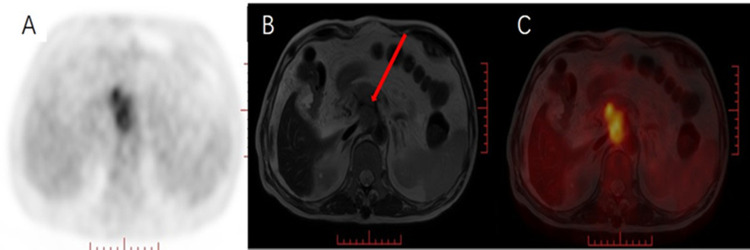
Male, 69 years old, with pancreatic cancer. (A) PET MIP image, heterogeneous hypermetabolism was seen in the pancreatic head area, (B) MRI T2WI pancreatic head space-occupying boundary was unclear, showing uneven isointensity, (C) PET/MRI fusion images of, and, respectively, pancreas Compared with the pancreatic background, the mass in the head area is inhomogeneous and hypermetabolic. Pancreatic head lesions compress the hepatic artery, but the course of the hepatic artery is clearly visible (red arrow).
